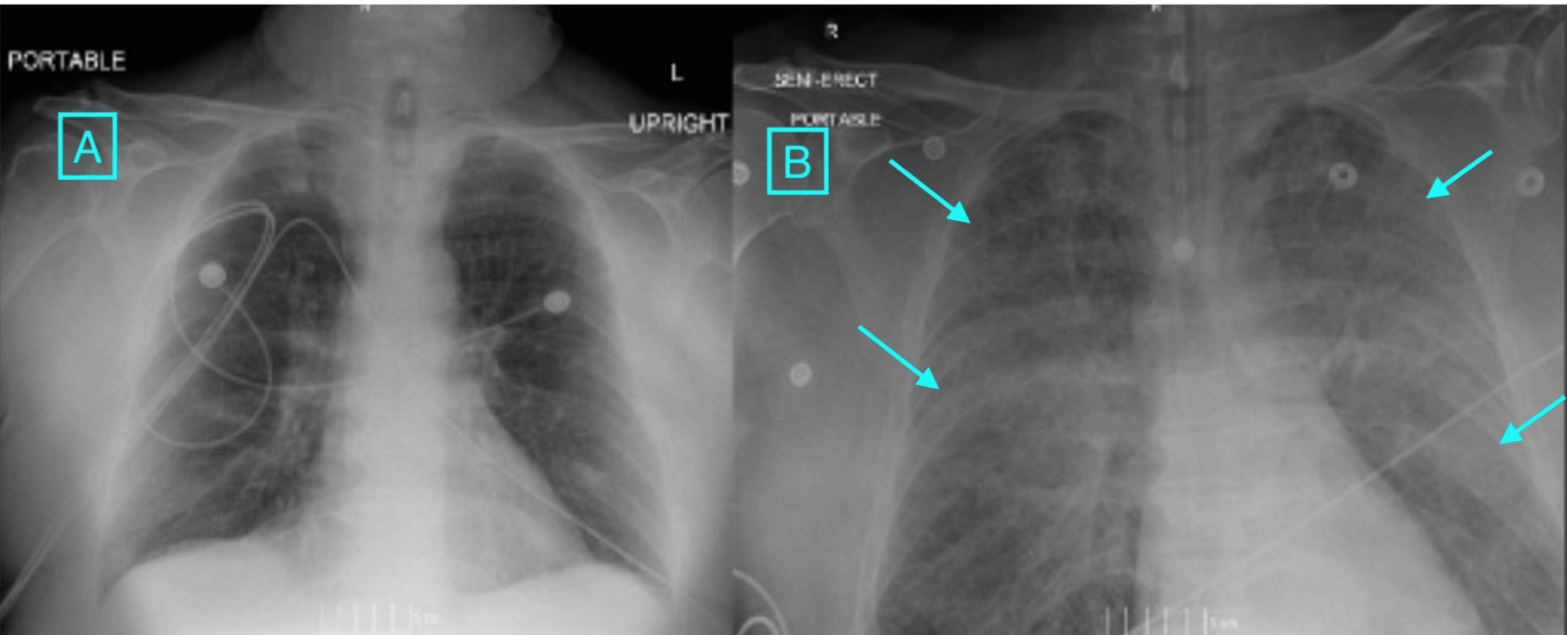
Chest X-ray showing the development of bilateral infiltrates (arrows) post-transfusion (B) when compared to pre-operative (A) with clear lungs
